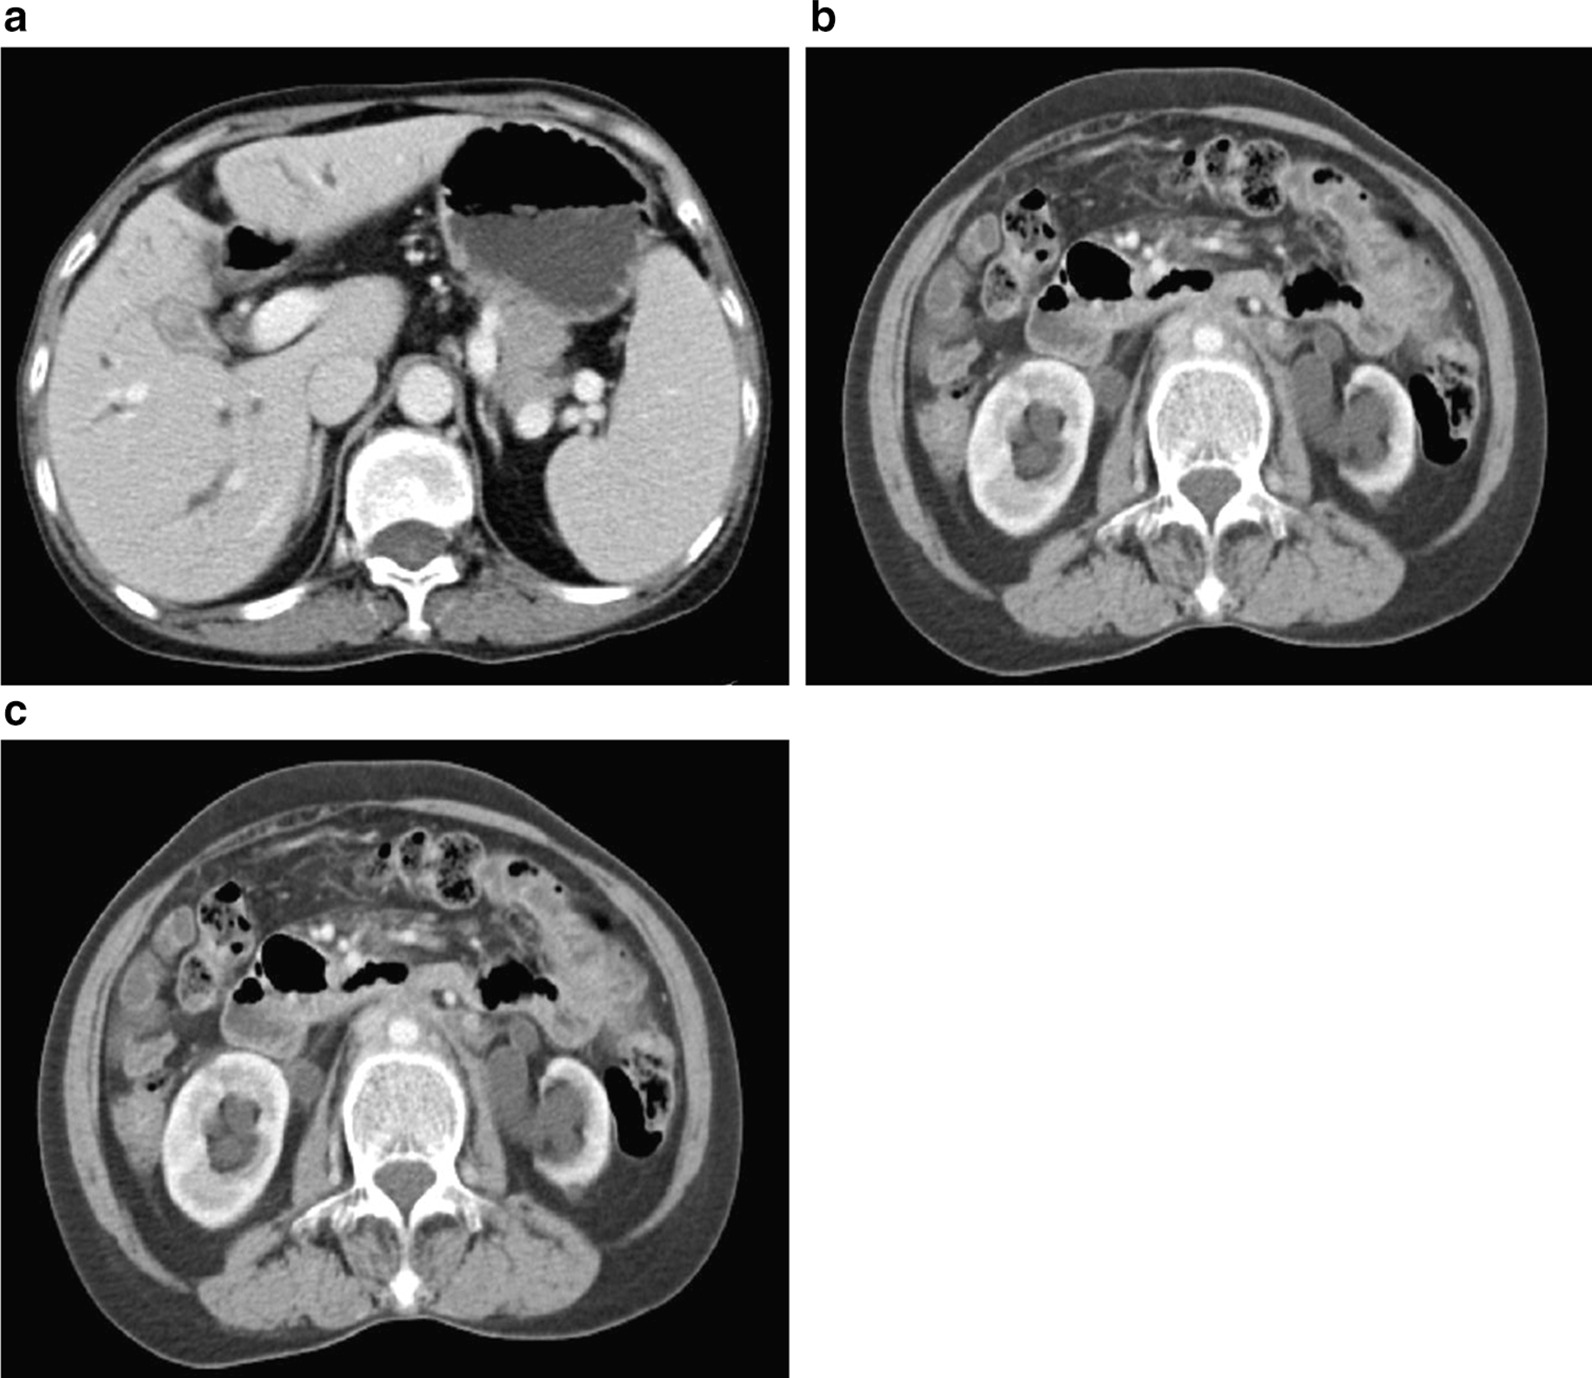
Characteristics of the abdominal CT scan. Abdominal computed tomography scan showing the gallbladder occupying and involving the hilar bile duct and dilatation of the proximal biliary system (a); posterior peritoneal mass occupying/involving the upper part of the bilateral ureter (b), and the change in the proximal urinary tract (c)
